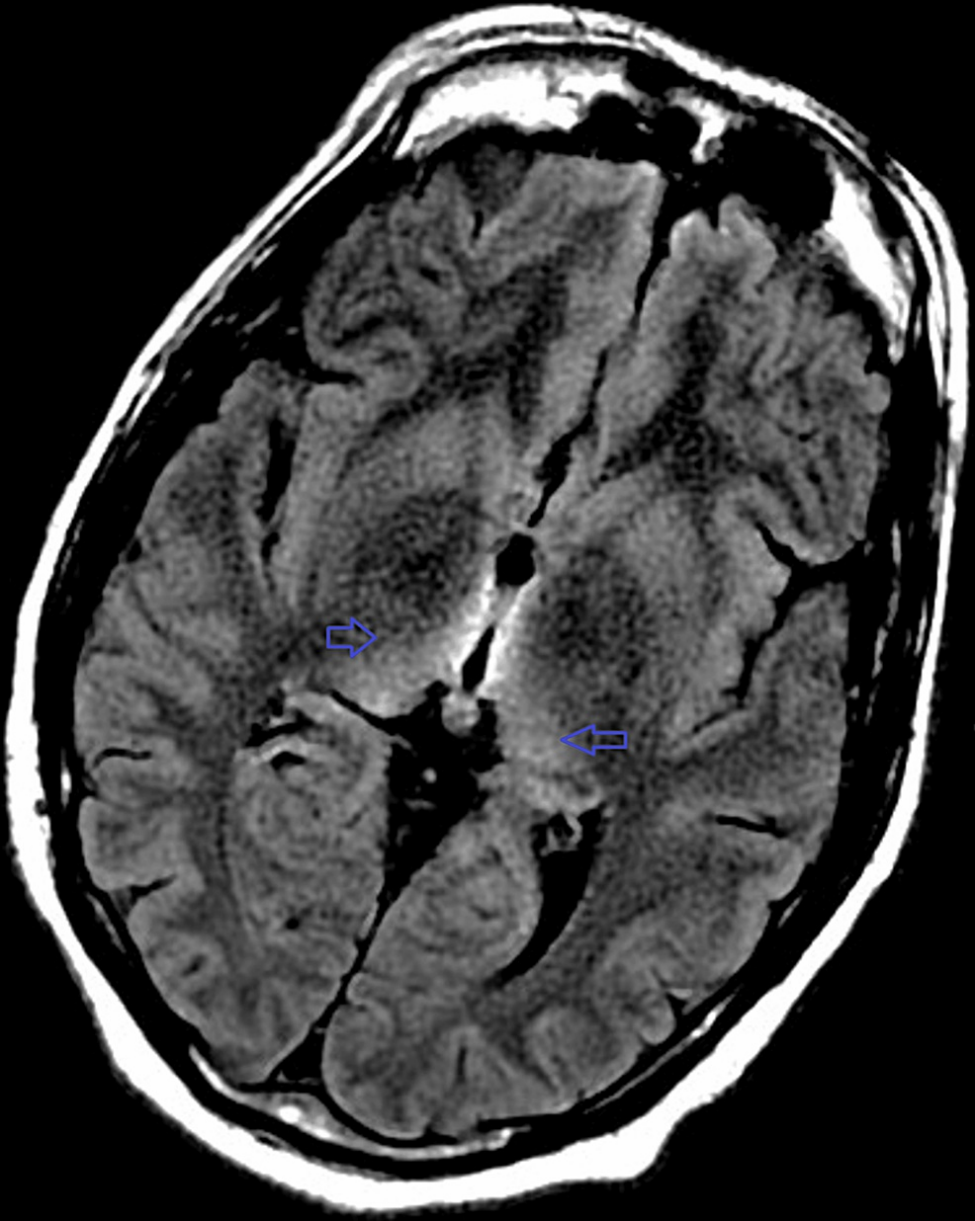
Typical MRI findings of Wernicke's encephalopathy. Bilateral pulvinar signal hyperintensity - hockey stick sign in Wernicke's encephalopathy (arrows).
